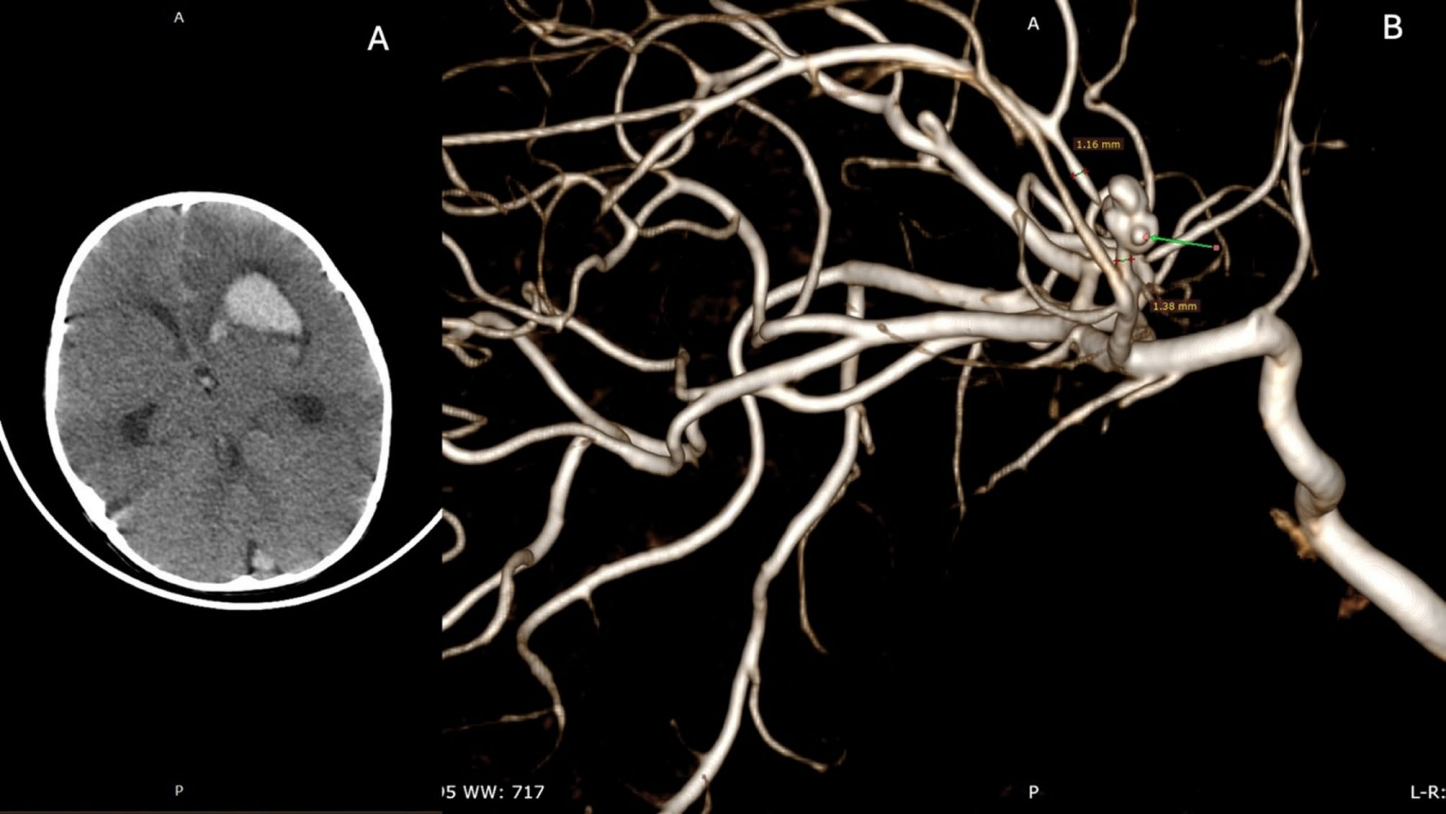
A: Preoperative CT demonstrates left frontobasal hematoma extended to ventricles B: After CT angiography revealed that an Acom aneurysm with multiple blebs is the cause of the parenchymal hemorrhage
